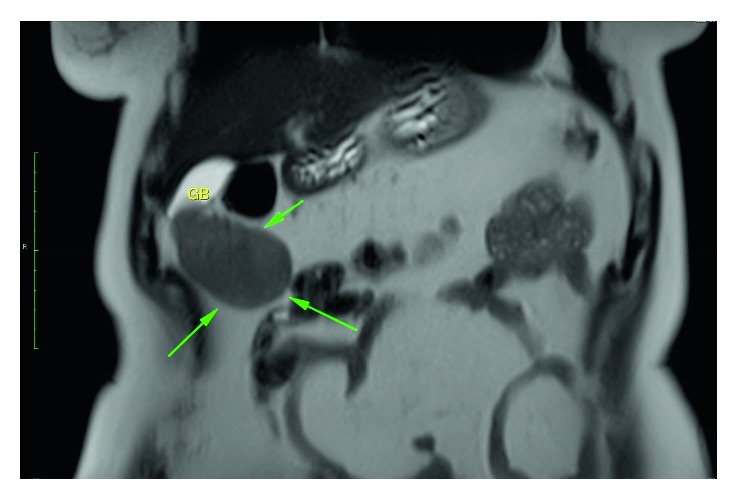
Coronal T2-weighted image. The mass extending from the gallbladder (arrows) and the gallbladder (GB) are seen.
